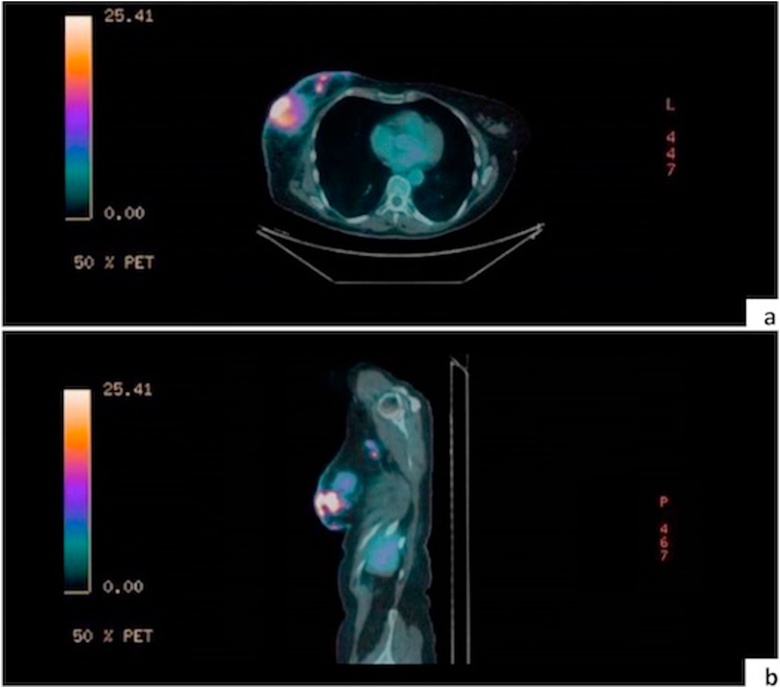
a) Axial PET-CT shows an intense hypermetabolism in right breast. b) Sagittal PET-CT shows an intense hypermetabolism in right breast and right axilla.
